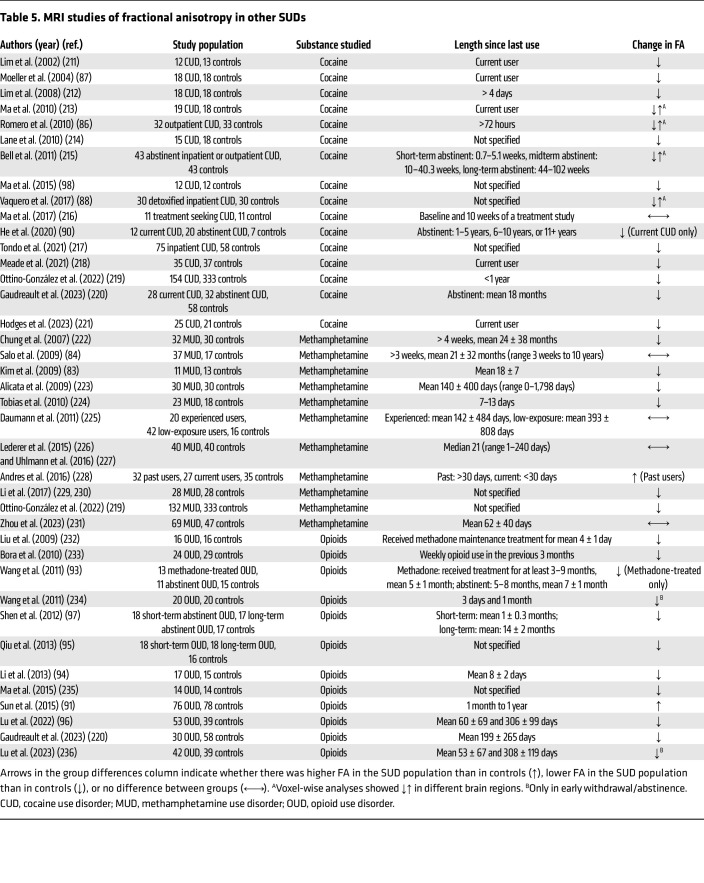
MRI studies of fractional anisotropy in other SUDs
